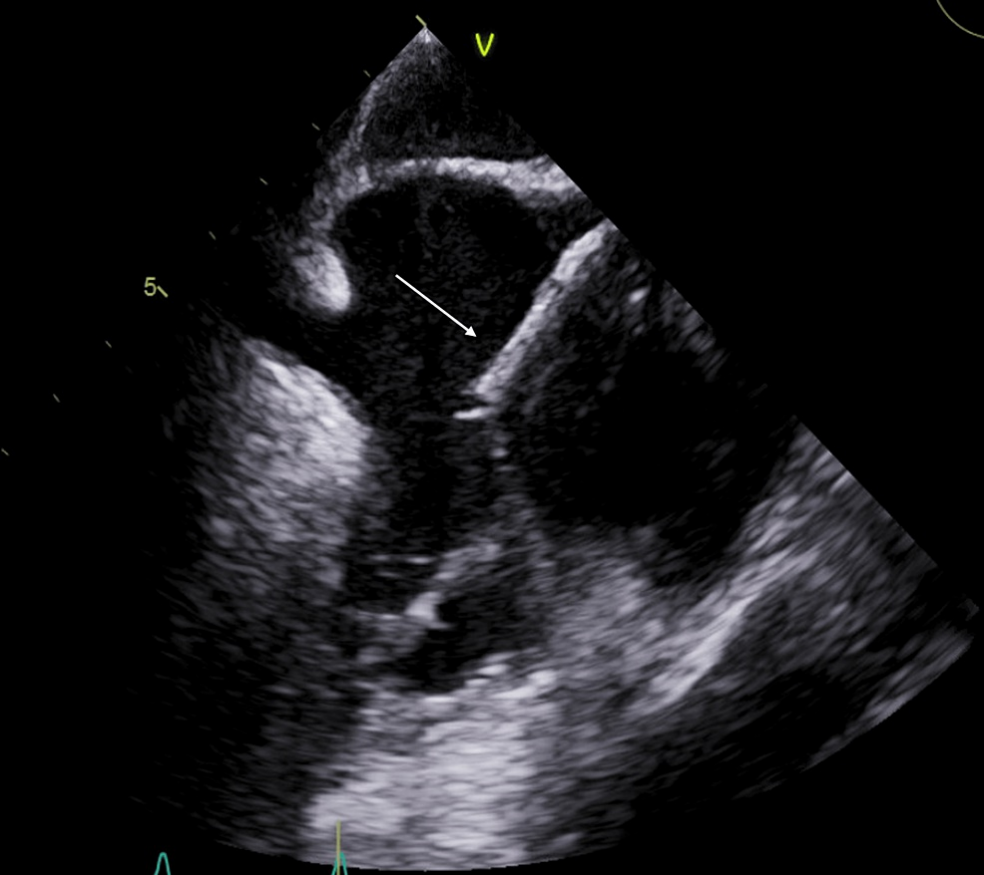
Transesophageal echocardiogram bicaval view. The image shows a calcified cast in the super vena cava entering the right atrium.
